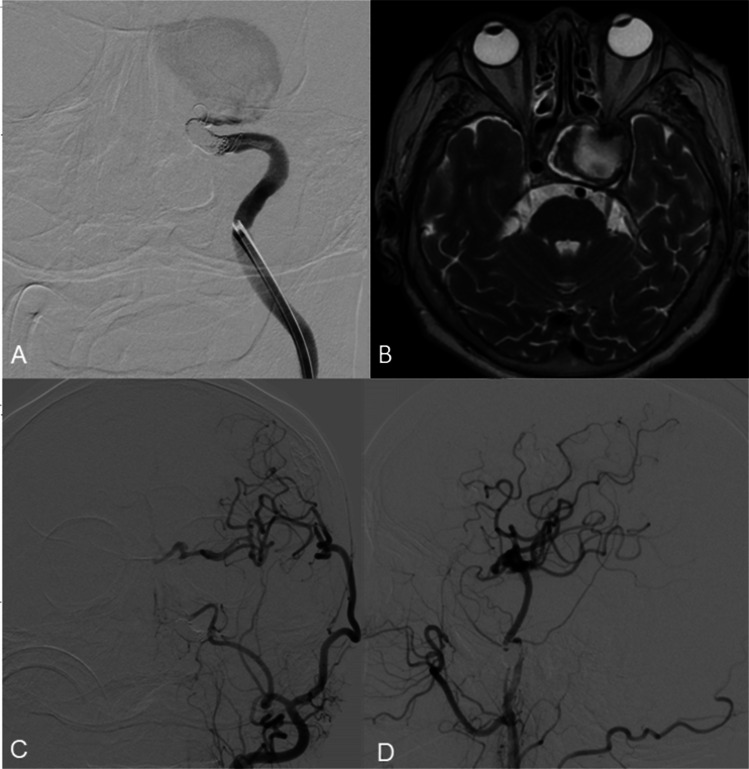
DSA from the left ICA (A) shows the coiled proximal and distal parts of the ICA aneurysm. DSA from left CCA (C, D) shows complete aneurysm occlusion and satisfactory patency of the double STA-MCA bypass after 3 months of follow-up. Three-month MRI (B) shows reduced aneurysm size and volume
